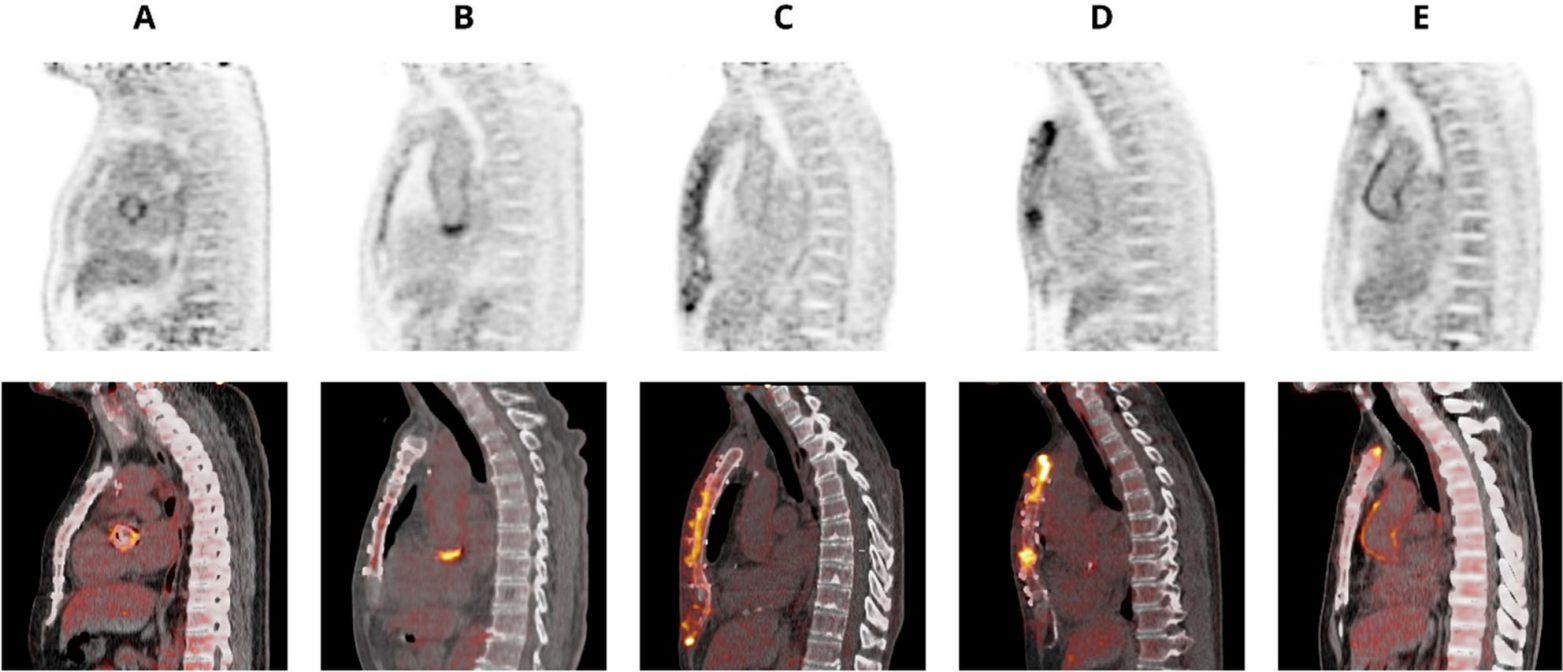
Examples of different categories of sternal ^18^FDG uptake patterns post-sternotomy. (A) no elevated uptake; (B) low-grade diffuse uptake; (C) high-grade diffuse uptake (D); high-grade heterogeneous uptake; (E) focal uptake
